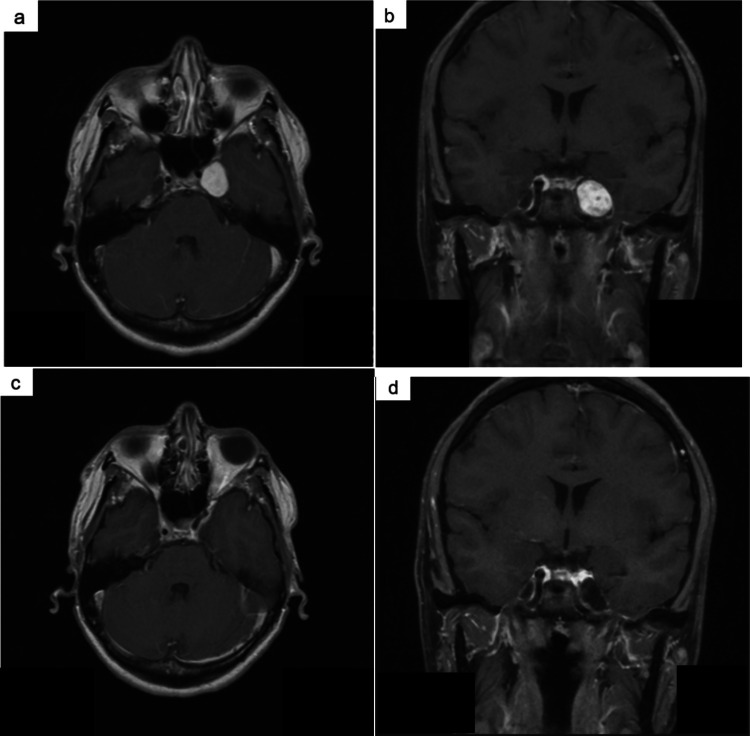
Preoperative axial (a) and coronal (b) T1 contrast brain MRI shows an enhancing left cavernous sinus lesion consistent with a trigeminal schwannoma. Postoperative axial (c) and coronal (d) T1 contrast brain MRI demonstrating complete resection of the lesion
